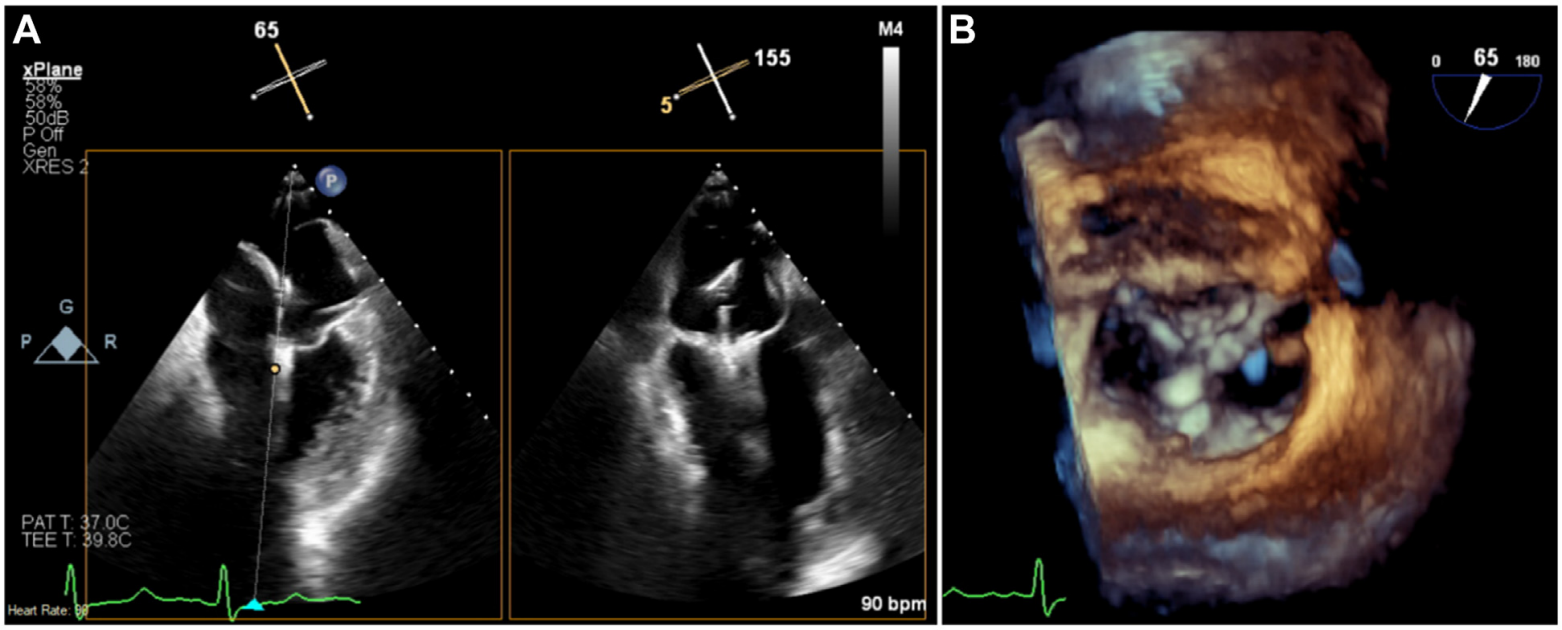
Predeployment Mitral Commissural View Successful capture of leaflets (A) followed by successful delivery of the MitraClip and visualization on 3-dimensional echocardiography (B).
